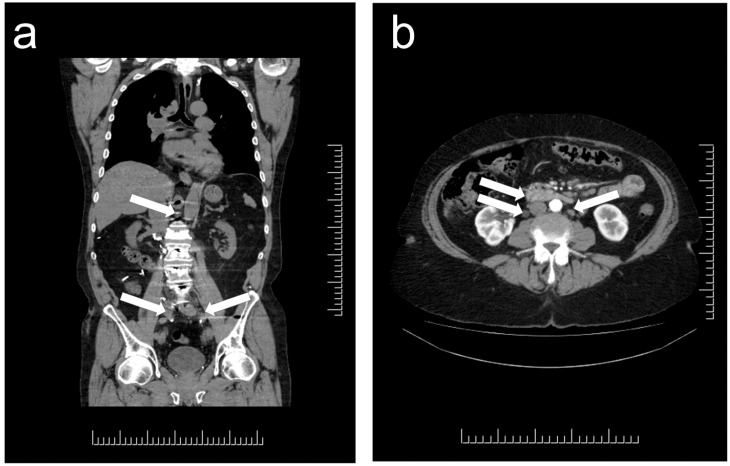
Localization of paragangliomas on Computed Tomography (CT) imaging. Multifocal paraganglia in patients with genetic predisposition to paraganglioma syndrome. (a) There are multiple small lesions all along the para-aortic channel (arrows), each representing a small paraganglioma in this patient with germline predisposition and multiple tumors, including multiple liver lesions. (b) In this patient with genetic predisposition to paraganglioma, there are multiple masses (arrows) that the radiology report identified as “multiple mesenteric lymph nodes, the largest one measures 5.1 × 1.9 cm, and there also sub-centimetric retroperitoneal lymph nodes”.
